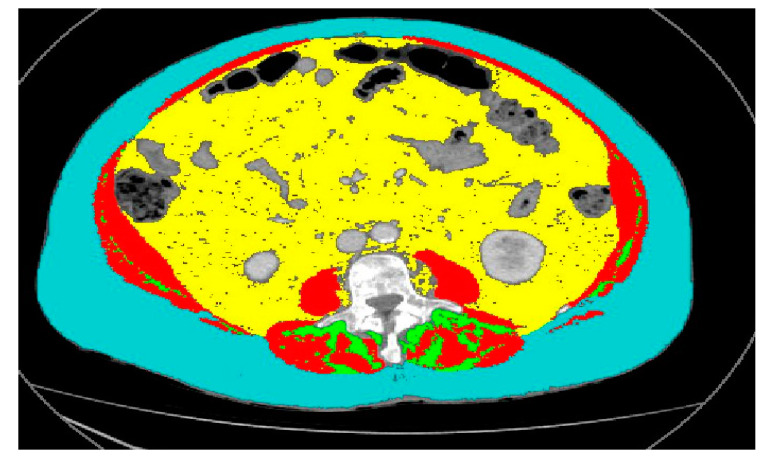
Example of PET/CT body composition analysis. Red: Muscle Mass; Blue: Subcutaneous Adipose Tissue; Yellow: Visceral Adipose Tissue; Green: Intramuscular Adipose Tisue.
